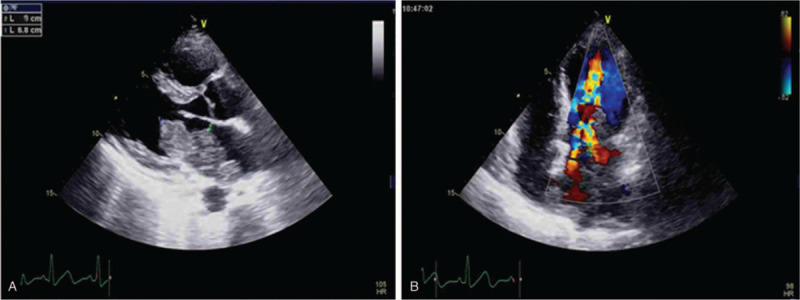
(A) Cardiac image section 4 cavities of an echocardiography: a large, irregular mass located on the lateral wall of the left atrium; (B) Color Doppler flow imaging (CDFI) shows a multicolored high-speed blood flow signal during diastole as a typical sign for mitral stenosis.
